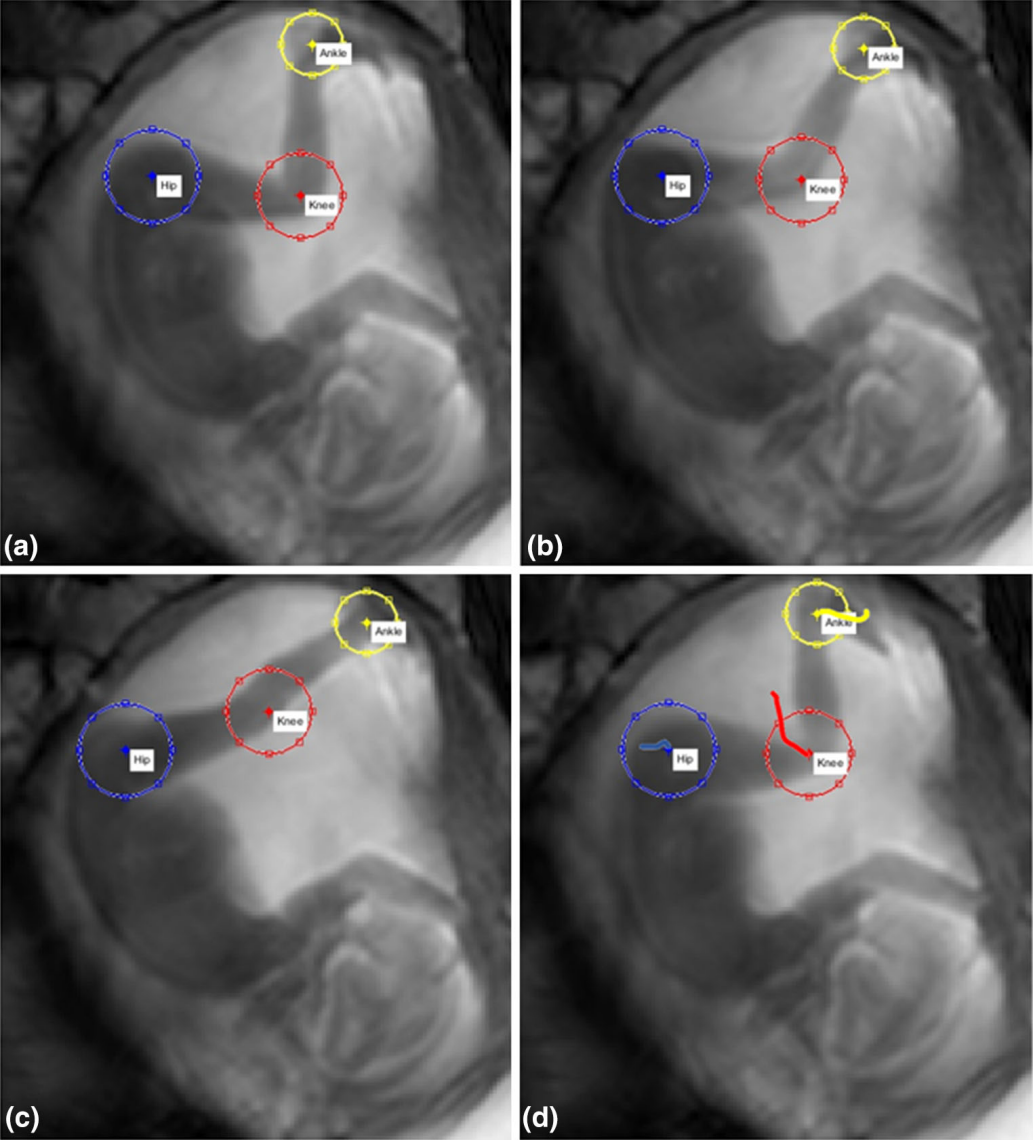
Successive frames (a-c) of cine-MRI scans, with (d) showing paths of displacement from automatic tracking of hip, knee and ankle joints using custom software
